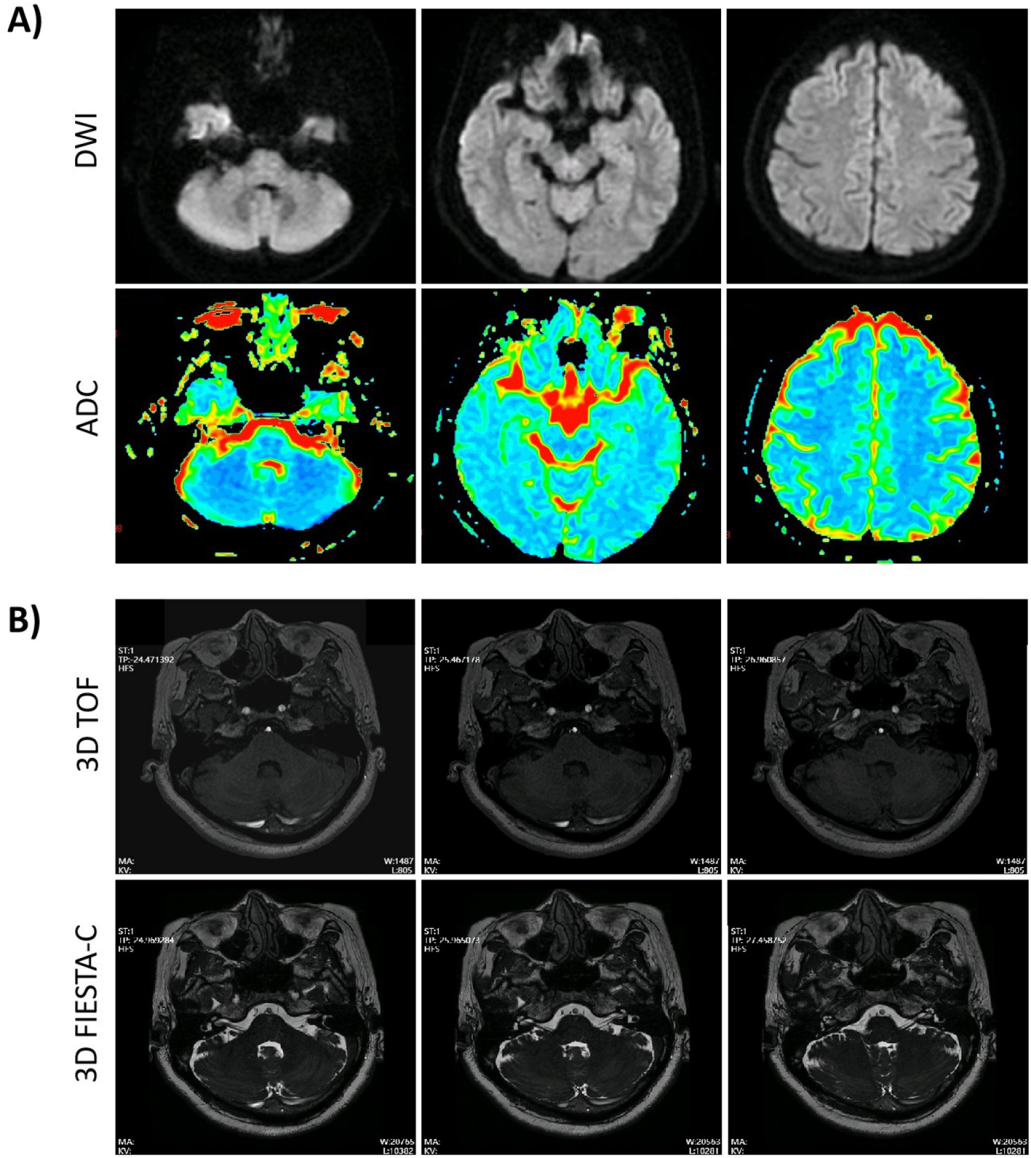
MRI findings. (A) Magnetic resonance diffusion-weighted imaging (MR-DWI) results obtained during the patient’s outpatient visit. No evidence of new cerebral infarction is observed (the upper row displays Diffusion-weighted imaging (DWI) sequences, and the lower row shows Apparent diffusion coefficient (ADC) maps). The three representative axial sections presented here correspond, from left to right, to the level of the internal auditory canal (including the vestibular nucleus), the cerebral peduncles, and the superior paracentral lobule. (B) High-resolution MRI of the facial and vestibulocochlear nerves acquired during hospitalization, showing no structural abnormalities. High-resolution images were acquired using an axial 3D FIESTA-C (Fast Imaging Employing Steady-State Acquisition with Cycled phases) sequence with a dual-excitation technique to minimize banding artifacts. MR angiography was performed using an axial three-dimensional time-of-flight (3D TOF) sequence.
